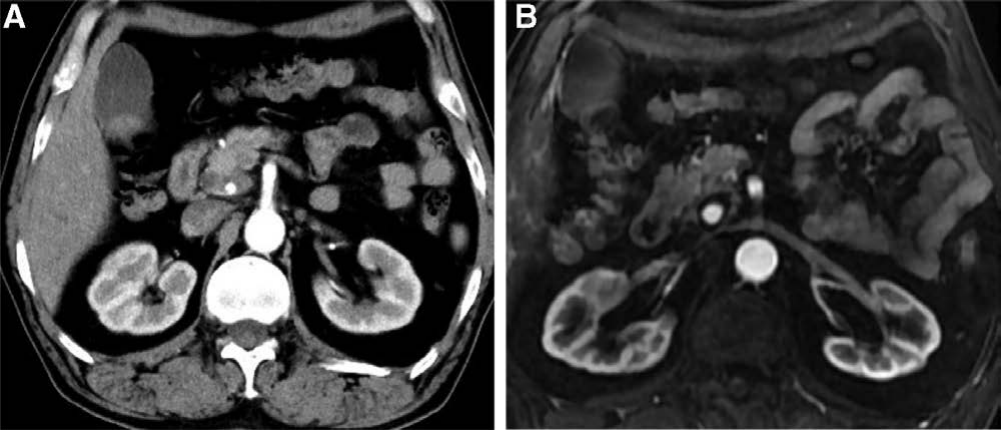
(A) Abdominal computed tomography suggested a possible pancreatic pseudoaneurysm. (B) Abdominal magnetic resonance imaging confirmed a pancreatic pseudoaneurysm.
